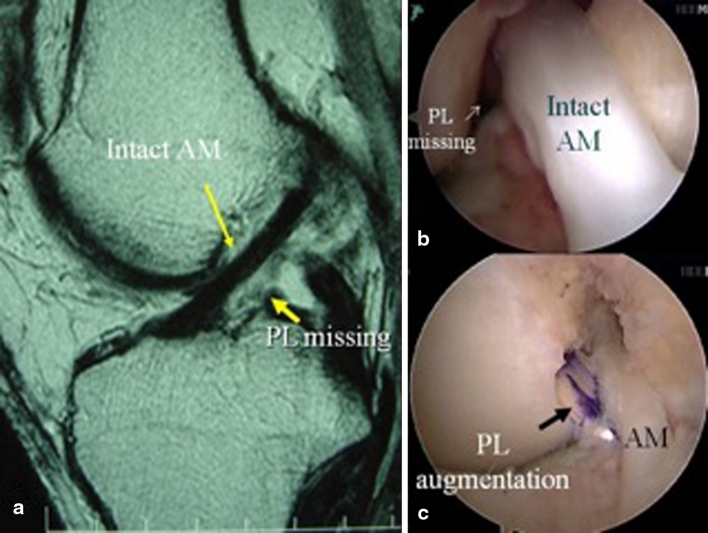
a MRI showing absence of PL bundle with AM bundle intact. b Arthroscopic picture confirming the absence of the PL bundle. c PL bundle augmentation
